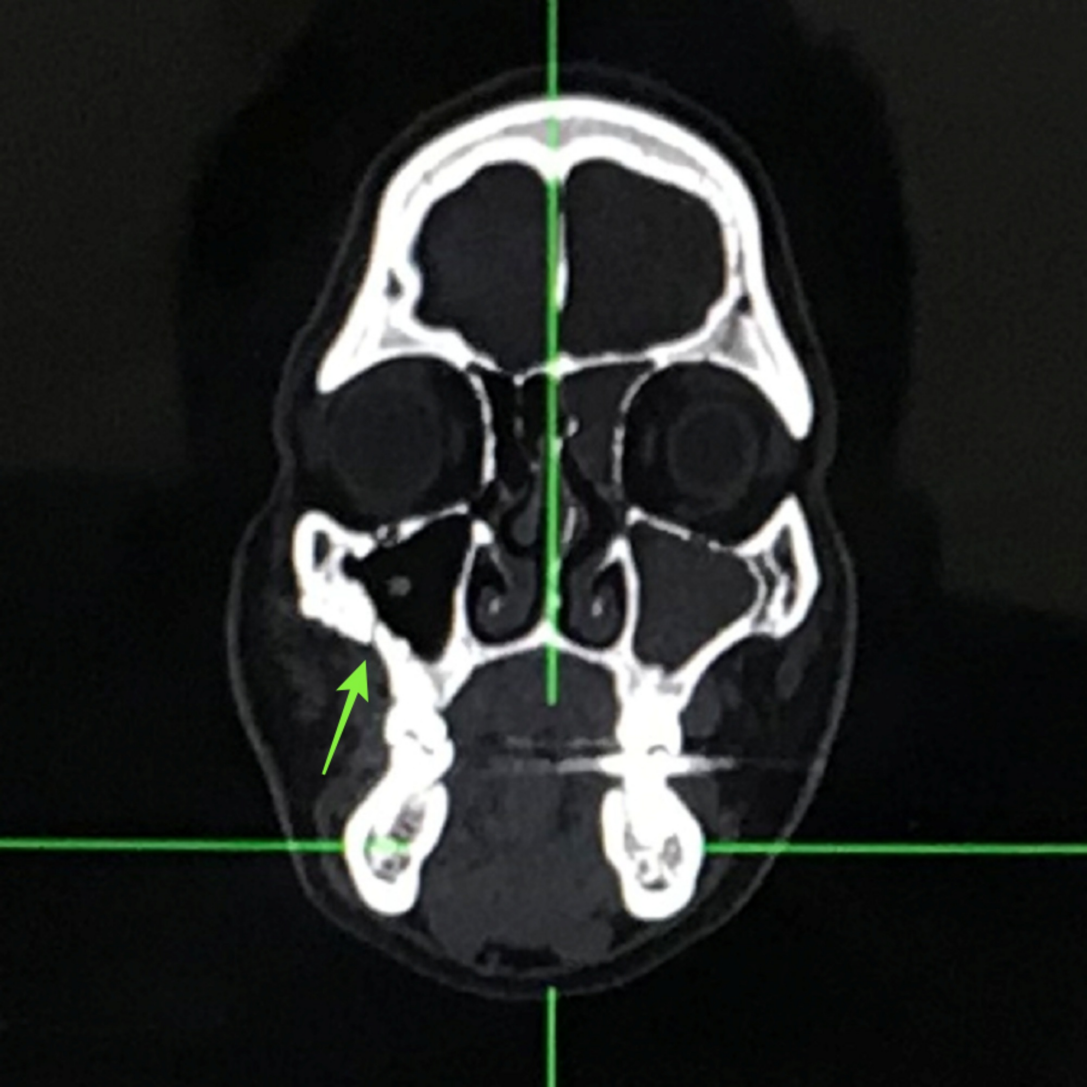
Postoperative computed tomography (coronal view) demonstrating acceptable fixation and lack of infection
